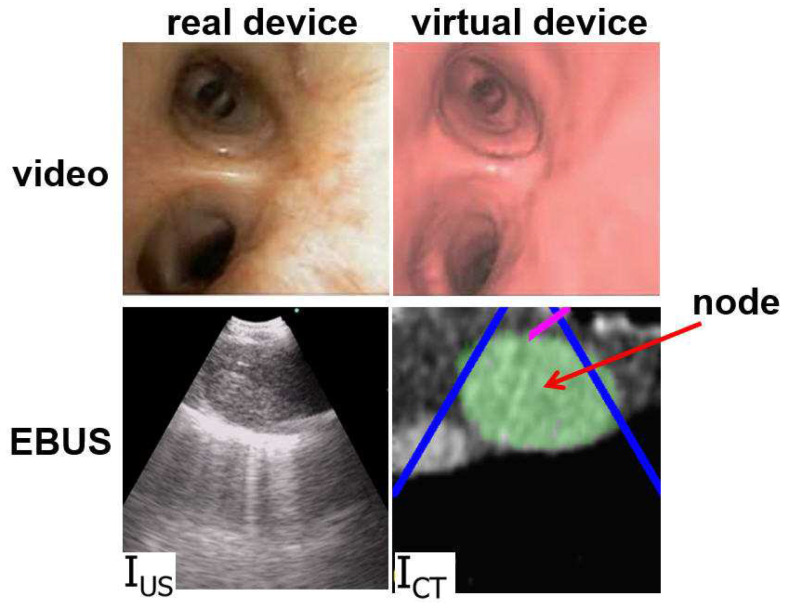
Example videobronchoscope and EBUS views constituting the multimodal EBUS bronchoscope. (Top) bronchoscopic video sources for the real EBUS bronchoscope and virtual device. (Bottom) corresponding fan-shaped EBUS views, $I_{\mathrm{US}}$ and $I_{\mathrm{CT}}$, for the real and virtual devices, respectively. For virtual EBUS view $I_{\mathrm{CT}}$, the blue lines demarcate the EBUS FOV, the magenta line indicates the video camera’s viewing direction $n_{C}$, and the green region denotes a lymph node predefined in the chest CT scan. In this figure, the videobronchoscope and EBUS view pairs are not at the same site. In addition, both view pairs are registered.
